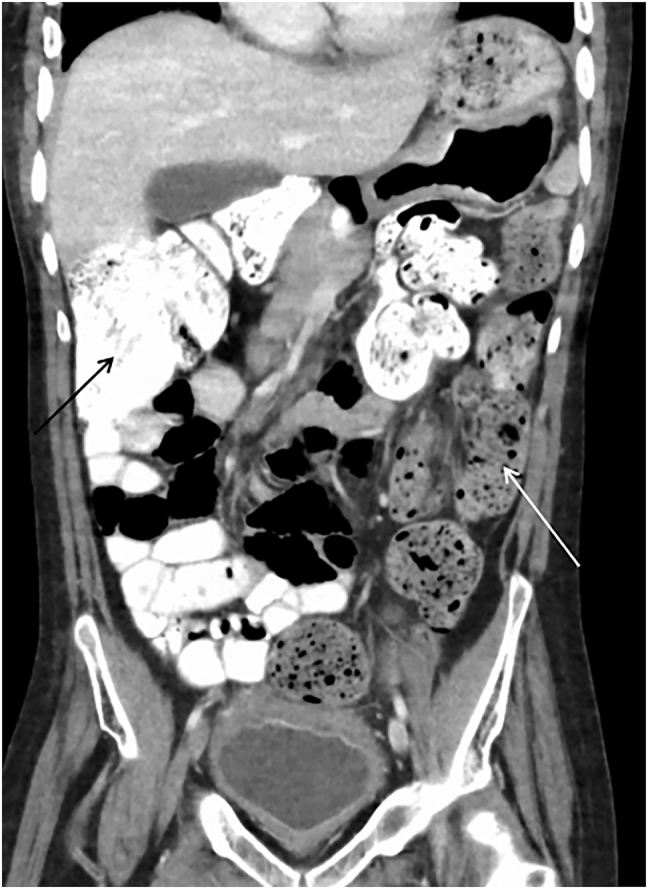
CT scan of the abdomen two years prior to the acute presentation, showing chronic dilatated and congested colon (thin arrows) without obvious mechanical obstruction, yet the presence of colonic dilatation up to 8 cm. Orally administered contrast medium was detected within the small intestine and ascending to the transverse colon but not in the descending colon.
